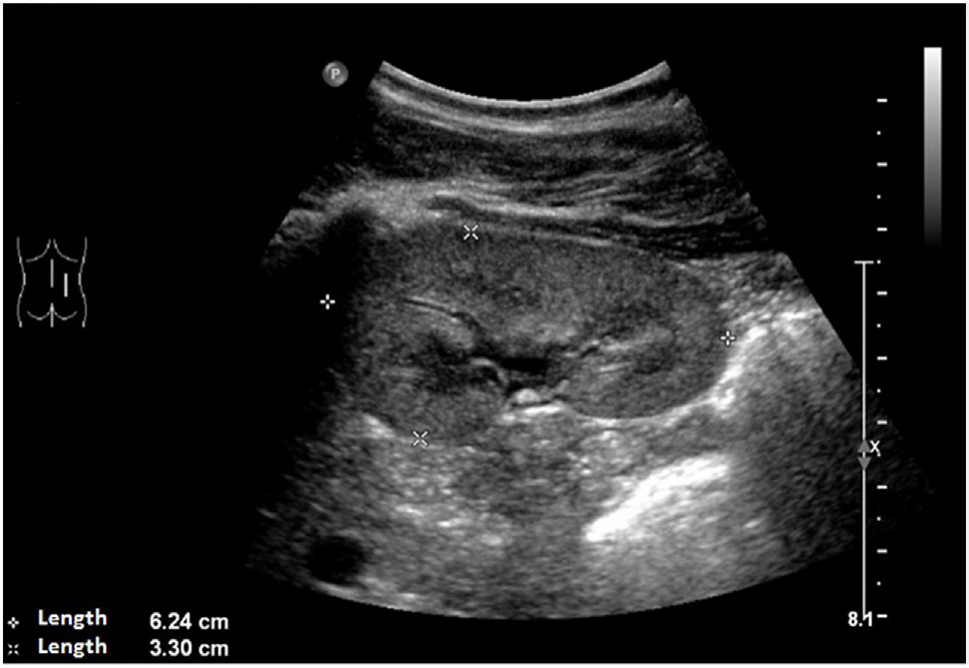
Ultrasound of the right kidney with a length of 6.2 cm (<1st percentile).
